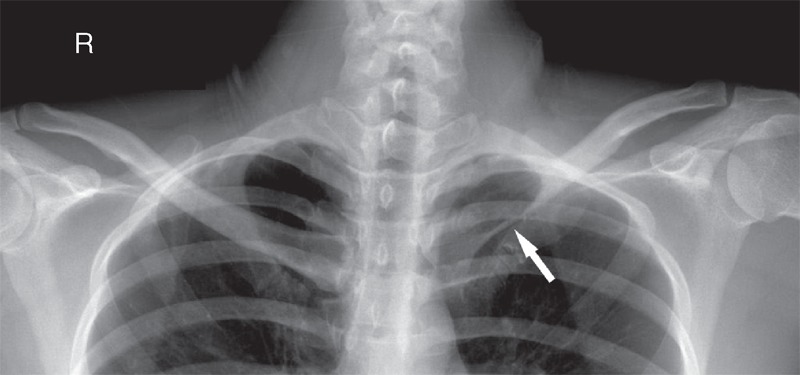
Posterior–anterior plain radiograph shows an ill-defined osteolytic lesion (arrow) without sclerotic margin in the medial part of left clavicle with soft-tissue swelling above the lesion.
